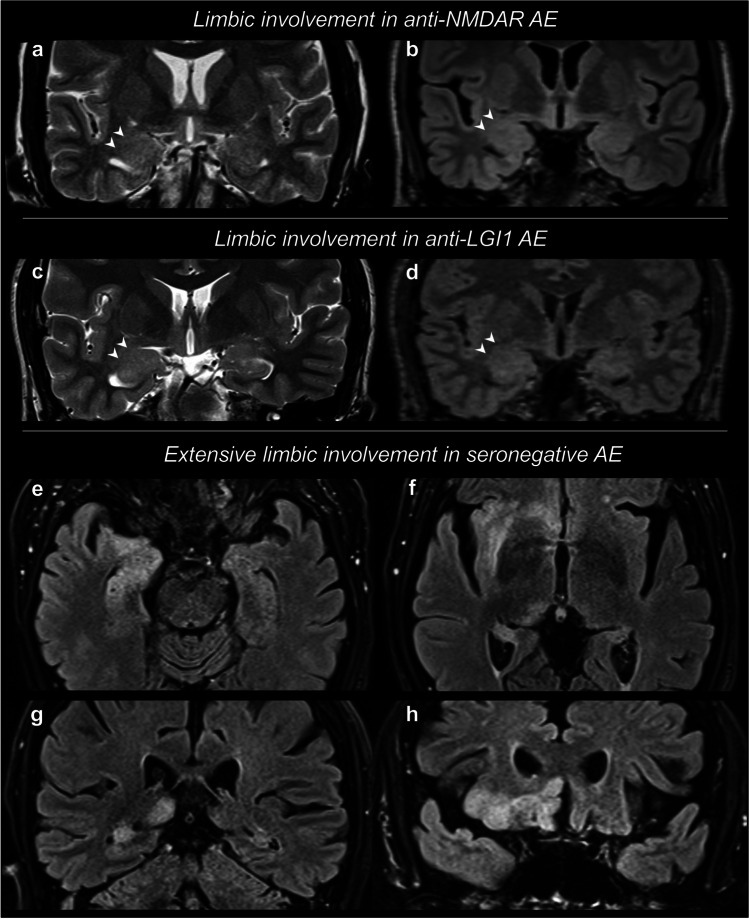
Limbic encephalitis. Limbic encephalitis (LE) can present with subtle radiologic findings, such as a swelling of the amygdala, with associated subtle signal hyperintensity, as visible (arrowheads) on coronal T2-weighted (a, c) and T2-weighted FLAIR (b, d) in two distinct cases of anti-NMDAR AE (a, b) and anti-LGI1 AE (c, d). Other patients may demonstrate more extensive alterations of the limbic structures. In this case (e–h), LE extensively involved the right limbic system, including amygdala (e), head of the hippocampus (e), body and tail of the hippocampus (e, f, g), insular cortex (f), thalamus/pulvinar (f), basal frontal gyri (f, h), and cingulate gyrus (h). White matter alterations are also noted, involving the external and extreme capsule (f). Following a thorough diagnostic work-up, a diagnosis of seronegative AE was posed
